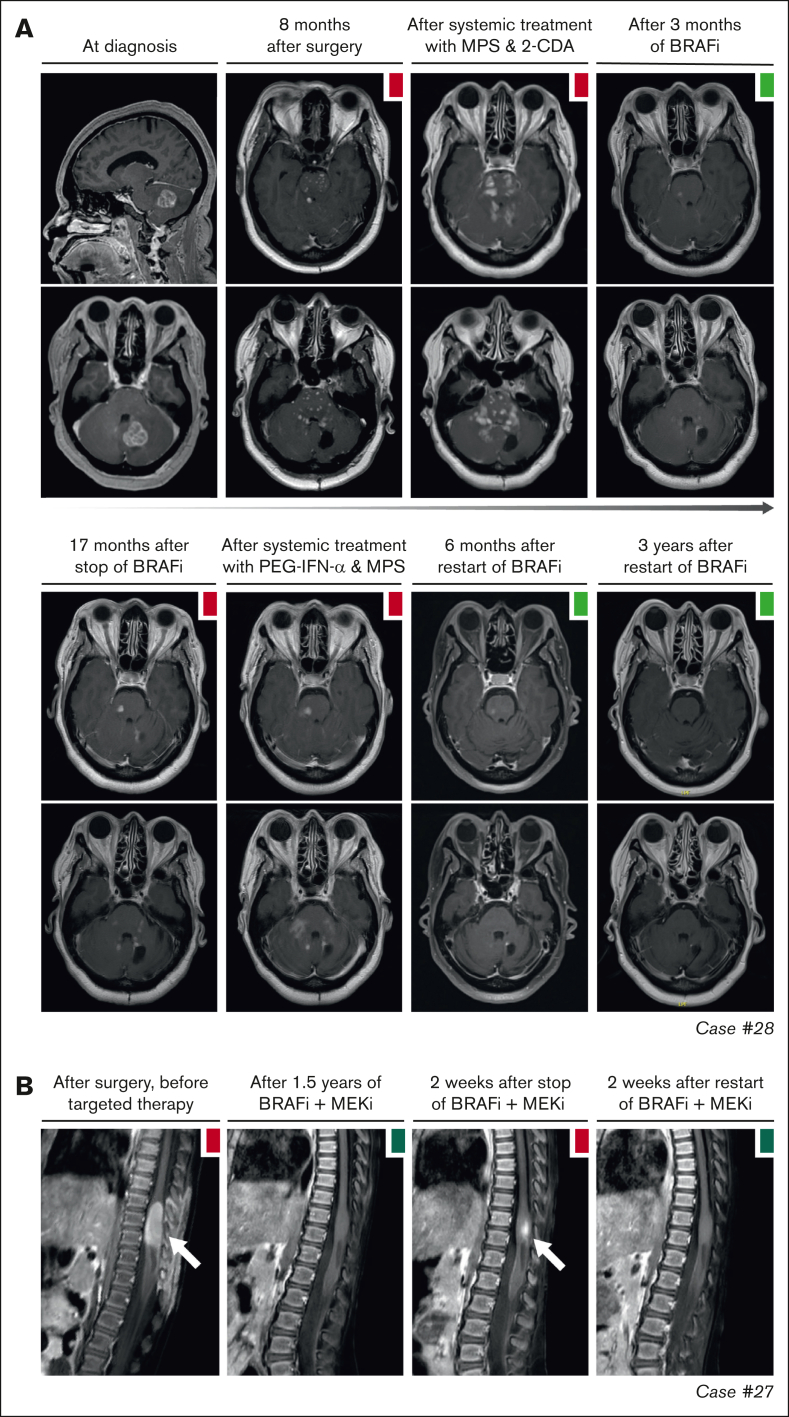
Disease progression after targeted therapy interruption. (A) Sagittal and axial MRI images of case 28, with CNS-AXG showing response of brain lesions to conventional therapies and targeted treatment with vemurafenib. After an initial PR to vemurafenib, targeted treatment was interrupted because of discontinued insurance coverage. Hereafter, brain lesions slowly progressed. Vemurafenib was restarted 3.6 years after interruption and quickly resulted in a decrease of brain lesions, with almost no lesions discernible at last follow-up. (B) Sagittal MRI images of case 27, with CNS-JXG showing relapse of a contrast-enhanced intramedullary spinal cord tumor after targeted therapy interruption. In this young child, treatment with dabrafenib and trametinib was interrupted after 1.6 years of therapy, while in complete radiologic remission. As an exit strategy, trametinib was stopped first, followed by dabrafenib 2 weeks later. MRI after stop of trametinib revealed persistent radiologic CR. However, MRI 2 weeks after stop of dabrafenib revealed recurrence of contrast-enhancement, consistent with a relapse of disease. MRI 2 weeks after restart of dabrafenib and trametinib showed that the radiologic response was recaptured. JXG, juvenile XG; MPS, methylprednisolone.
